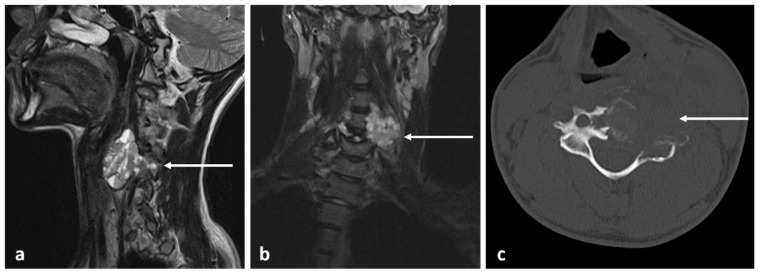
Sagittal T2 (a), coronal STIR (b) and axial CT (c) images of a cervical vertebral aneurysmal bone cyst (white arrows). The expansile lytic appearance on CT and multicystic appearance with fluid levels on MRI are typical features.
